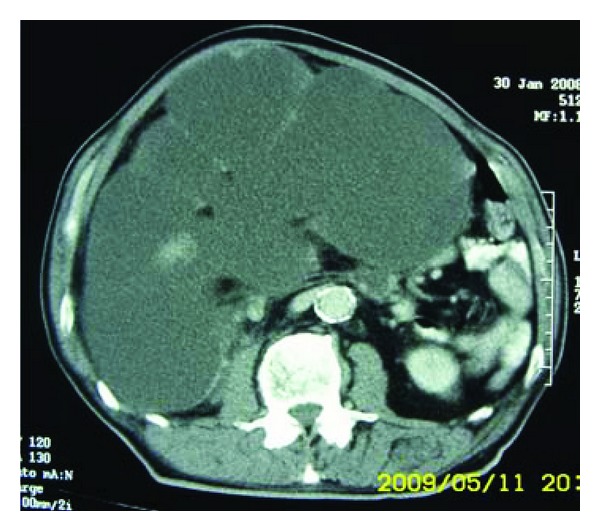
CT of the abdomen showing giant hydronephrosis.
